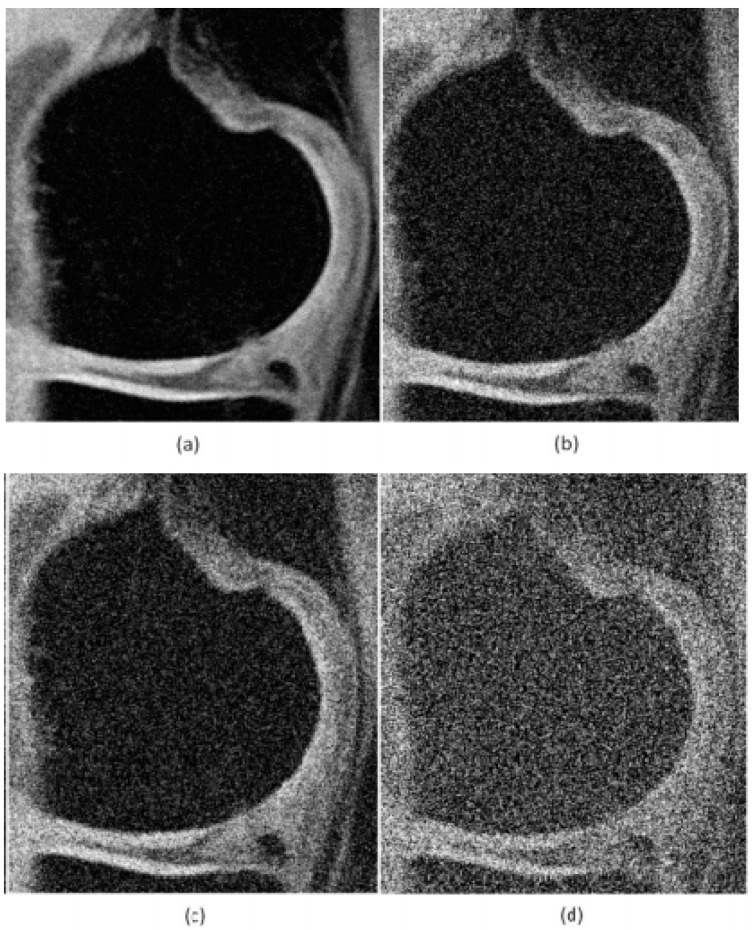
Selected area of interest (RoI) for MR image of articular cartilage with dynamic Rician noise intensity: (a) σ=0.02, (b) σ=0.09, (c) σ=0.15, and (d) σ=0.75.
